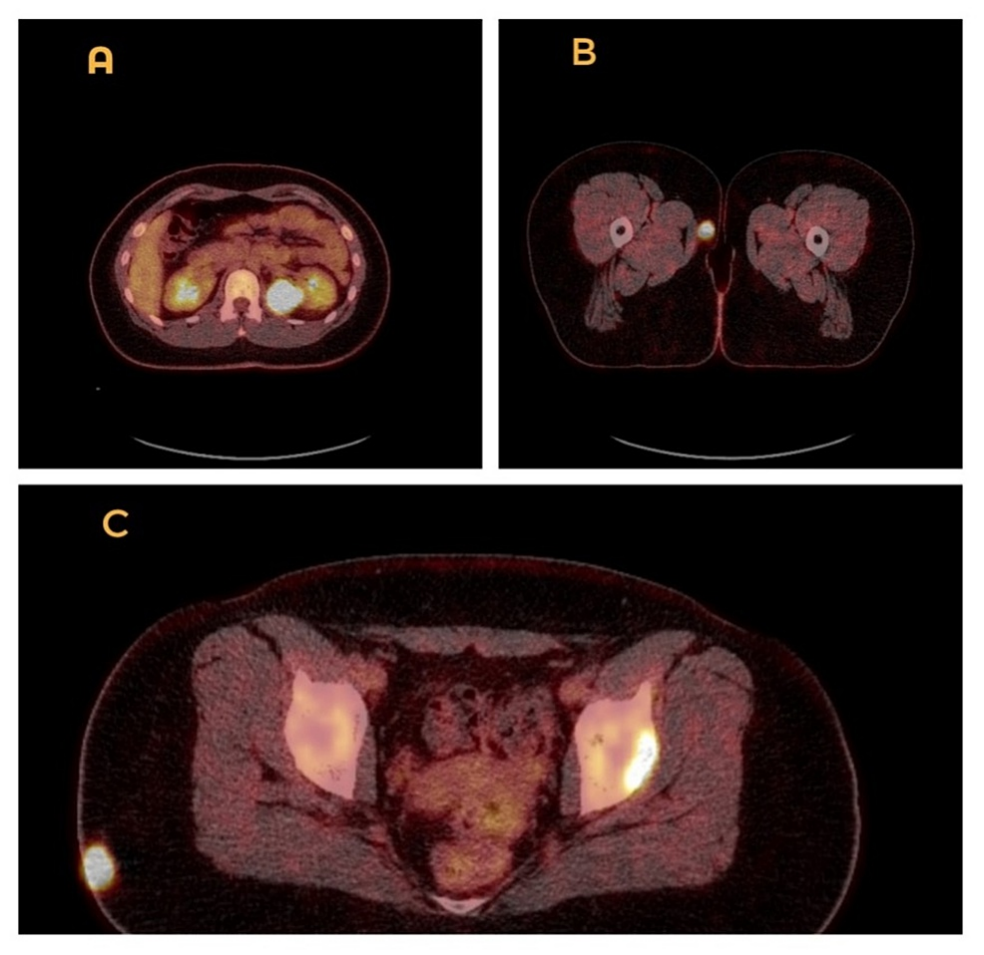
PET-CT scan showing fluorodeoxyglucose (FDG) avid lesions in the left kidney (A), subcutaneous area of the medial aspect of right upper thigh (B), left acetabulum as well as lateral aspect of the right buttock (C).
